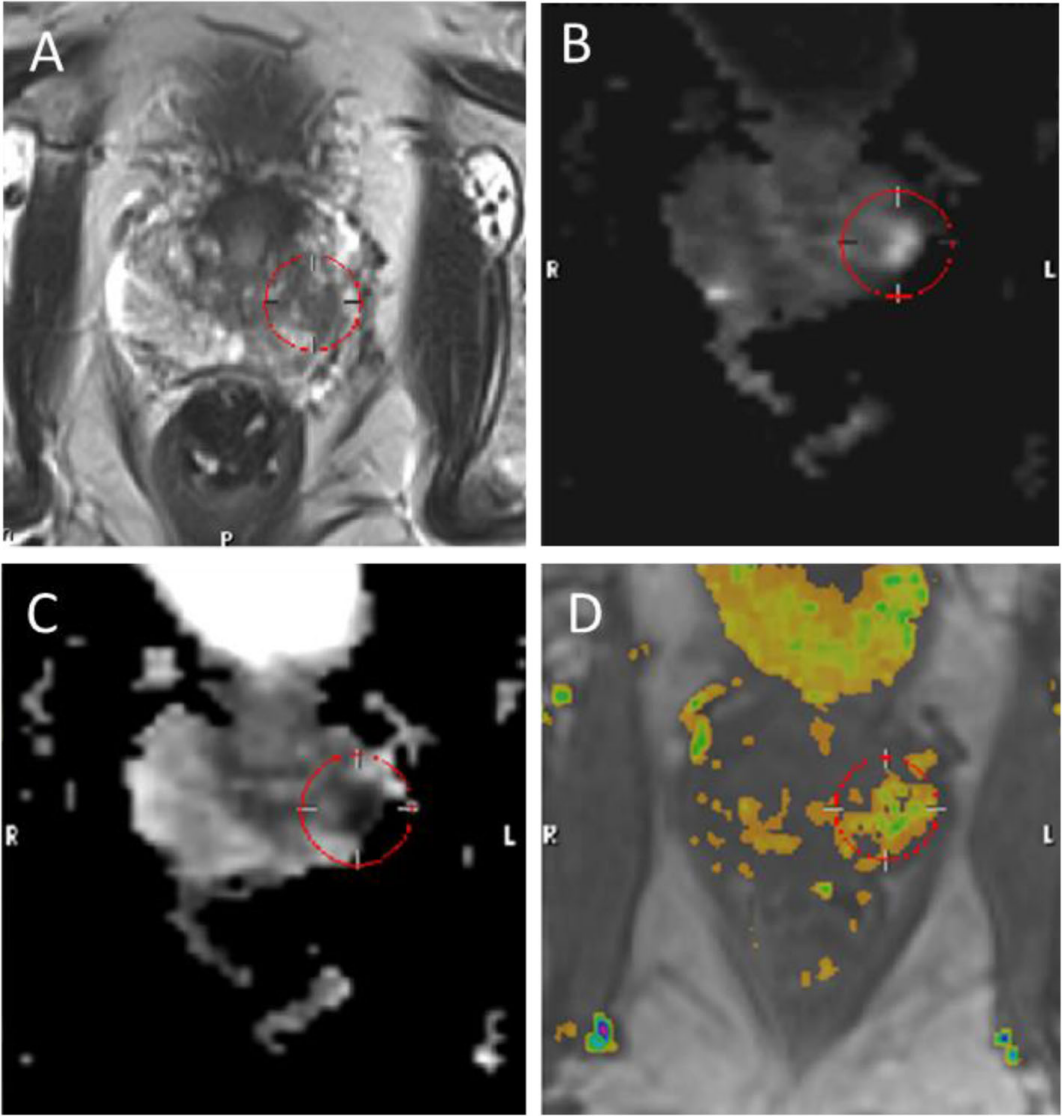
In November 2015, the patient of case 1 had a PSA level of 10.8 ng/ml and was referred for multiparametric prostate 3 T MRI for diagnostic purposes because the urinary biomarker-based risk score was 22% for high-grade PCa indicating the need for prostate biopsy. a An axial T2-weighted image shows a lesion on the left peripheral zone at the prostate base anterior side (red circle). b An axial diffusion-weighted image (DWI) shows an area of increased signal at the left peripheral zone. c An axial apparent diffusion coefficient (ADC) map shows a corresponding area of low ADC value (red circle). d A colorized perfusion map created from dynamic contrast-enhanced MRI (DCE-MRI) acquisition shows corresponding abnormal enhancement kinetics at the same location. All sequences identified the same region in the left anterior peripheral zone. The PI-RADS 5 outcome confirmed the biopsy indication of the urinary biomarker-based test outcome. TRUS-guided biopsies from that region confirmed high-grade Gleason 3 + 4 = 7 PCa
